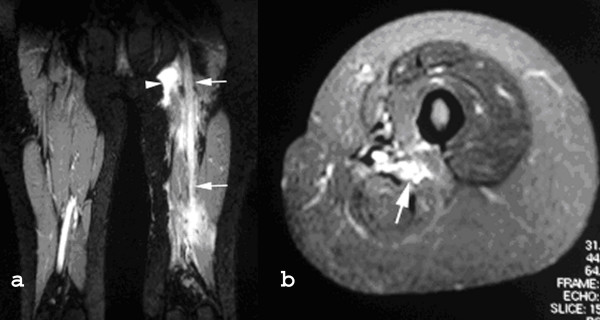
Follow up MRI of the thigh. Coronal STIR (a) and axial fat suppressed T2W FSE (b) images showing diffuse swelling and oedema of the sciatic nerve (arrows) and a postoperative seroma in the groin (arrowhead).
